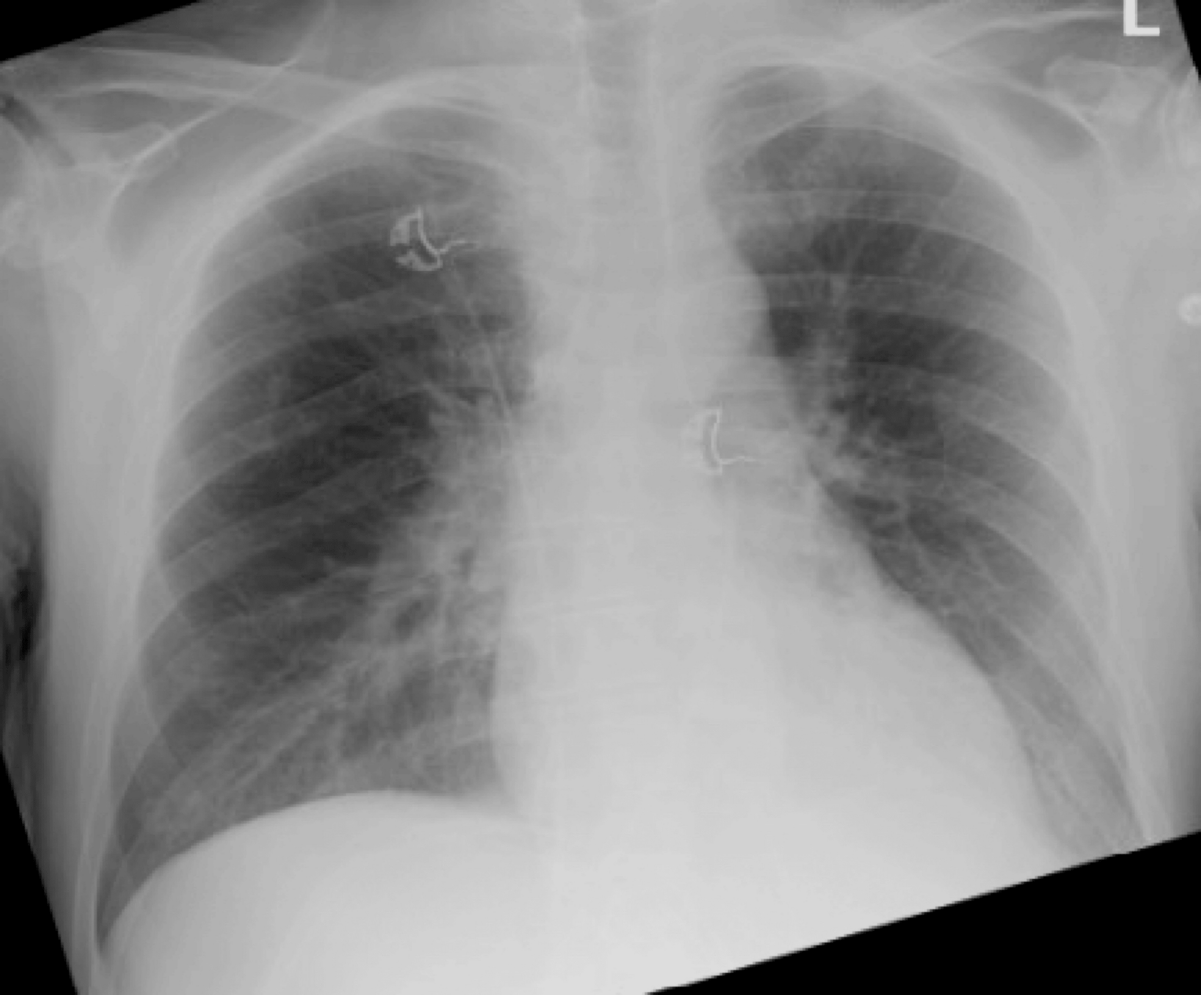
Chest radiograph (limited image quality) demonstrating pulmonary vascular cephalization, suggestive of congestive heart failure.
